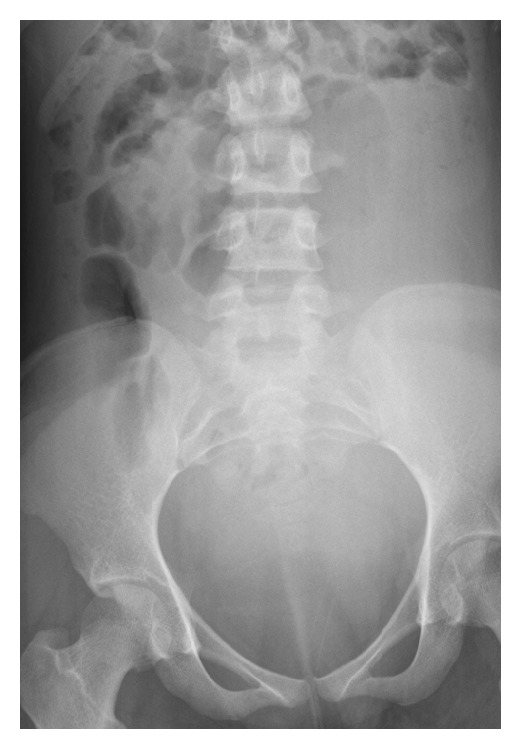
Abdominal radiograph demonstrating a large mass in the left lower quadrant, displacing bowel loops.
